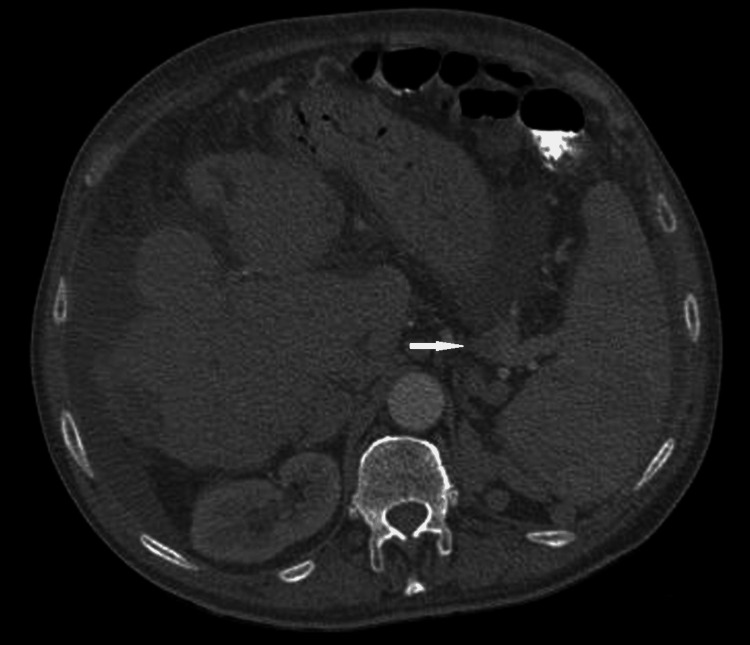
Abdominal CT scan showing splenic hilum varices (white arrow).
